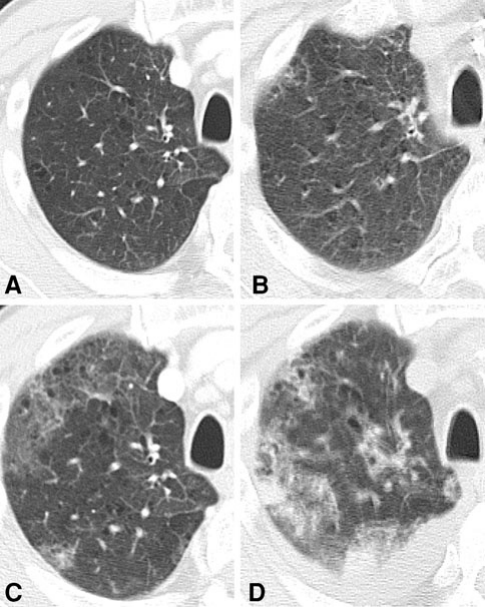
The progressive multiple lung shadows were detected with chest CT. Twelve days before admission (a), on the 2nd hospital day (b), 11th day (c) and 19th day (d)
